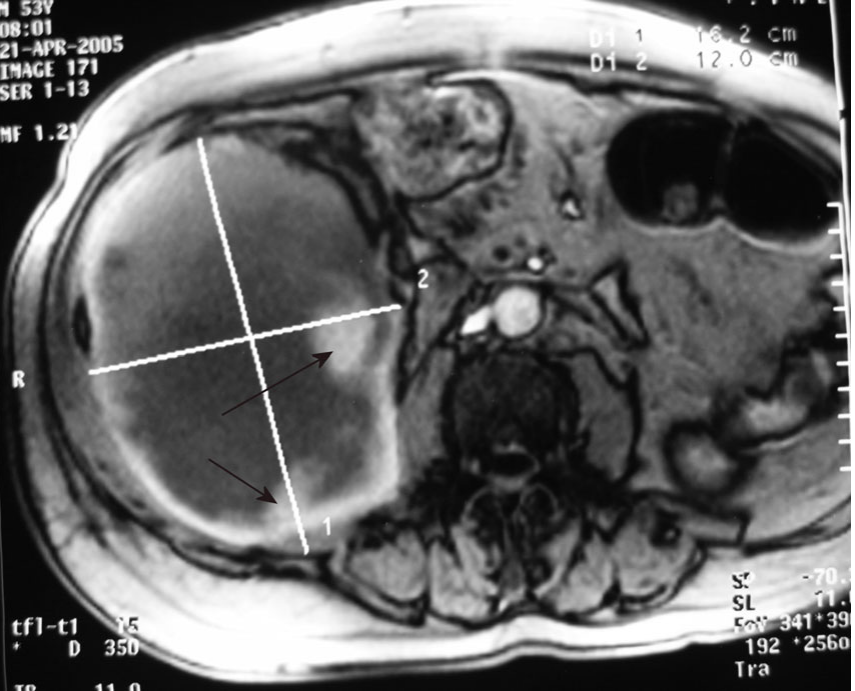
MRI – axial plan showing a large, mixed density mass in the right side of the abdomen suggestive of a large retroperitoneal hematoma, with areas of hyperdensity (arrows) indicating ongoing hemorrhage.
